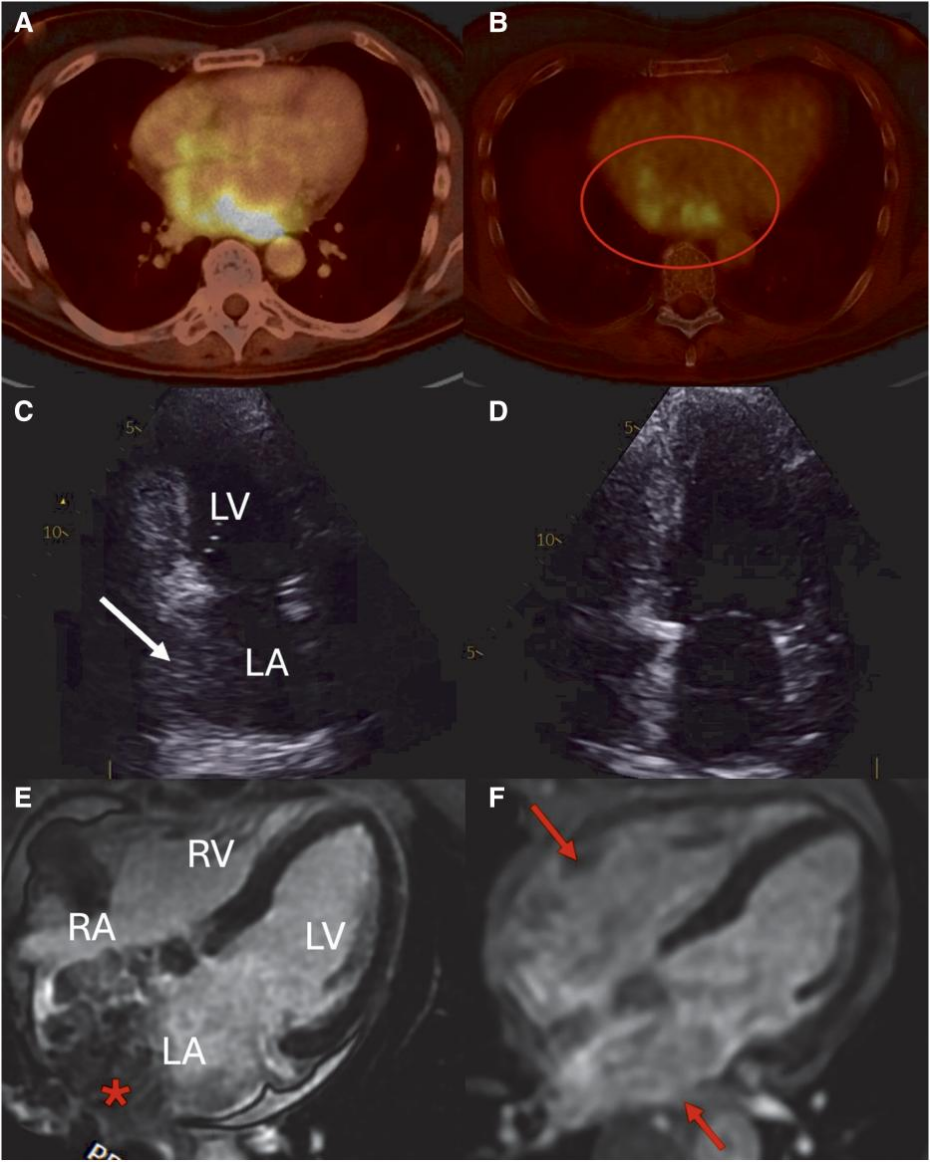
Comparison before and after treatment. Multimodal imaging demonstrates a marked reduction in cardiac mass size following systemic therapy. (*A–C*) Transthoracic echocardiography and cardiac magnetic resonance obtained before treatment show extensive infiltration of both atria and involvement of the right pulmonary vein. Positron emission tomography–computed tomography demonstrates intense fluorine-18 fluorodeoxyglucose (FDG) uptake within the cardiac mass (SUV_max 8), consistent with metabolically active tumour. (*D–F*) Follow-up imaging reveals significant tumour regression. Cardiac magnetic resonance shows residual thickening in the lateral wall of the right atrium (9 mm) and the inferior wall of the left atrium (6 mm). FDG uptake in the cardiac mass (posterobasal aspect of the left atrium) has decreased in both intensity and extent; however, residual metabolic activity persists (SUV_max 6).
